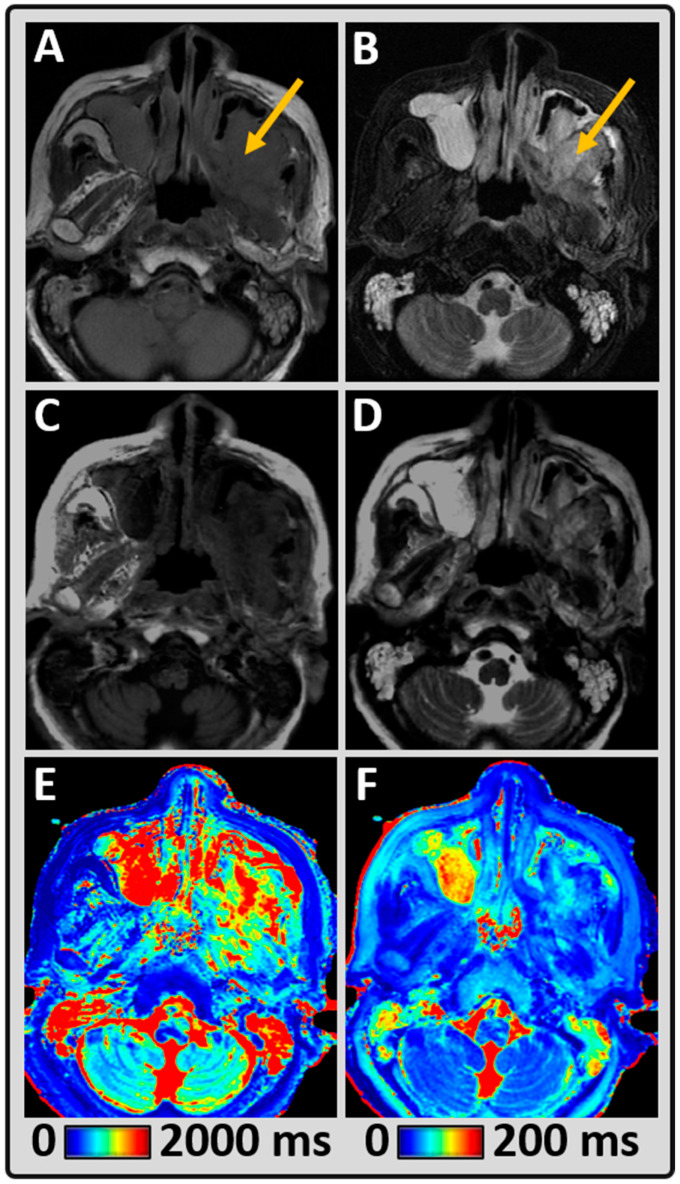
Representative MRI data from an 83-year-old female patient with yellow arrow pointing at left maxillary sinus secretory carcinoma of the salivary gland treated with radiation therapy. (A,B) Conventional qualitative T1w and T2w images demonstrating the tumor lesion; (C,D) Synthetically generated T1w and T2w images using MAGiC; (E,F) MAGiC-generated quantitative T1 and T2 maps.
